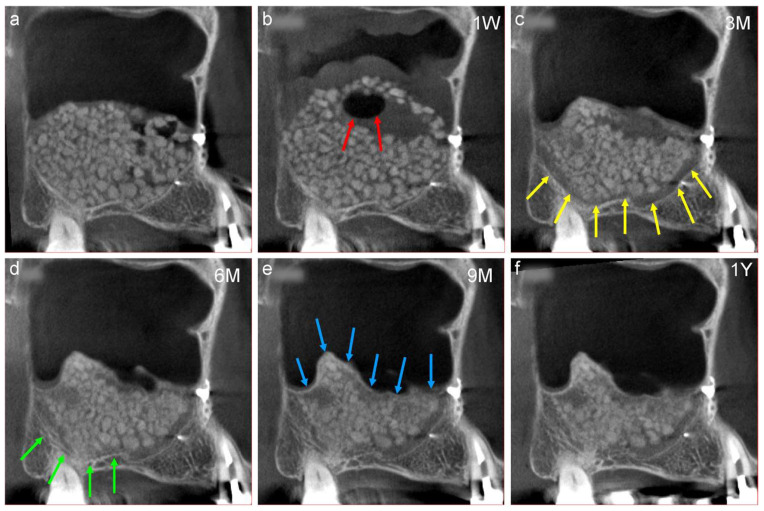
(a) Mesiodistal section of the CT image obtained after sinus floor elevation. Sufficient β-TCP granules are observed at the augmented area. (b) One week after surgery, the β-TCP granules moved upward owing to postoperative swelling of the sinus membrane. An air around β-TCP granules has accumulated at the upper portion of the augmented area (red arrows). 1W; one week follow-up (c) Swelling of the sinus membrane disappeared spontaneously three months postoperatively, and a belt-shaped radiolucency (grey zone) is observed at the interface between the β-TCP granules and the surface of the maxillary sinus (yellow arrows). 3M; three months follow-up (d) The radiopacity of the original cortical bone decreased six months postoperatively, and that of the grey zone increased (green arrows). 6M; six months follow-up (e) A complete radiopaque line (white line) is observed at the newly formed bottom of the maxillary sinus (blue arrows) nine months postoperatively. 9M; nine months follow-up (f) The white line becomes clear 12 months postoperatively; however, the β-TCP granules in the central area retained their shape. 1Y; one year follow-up.
